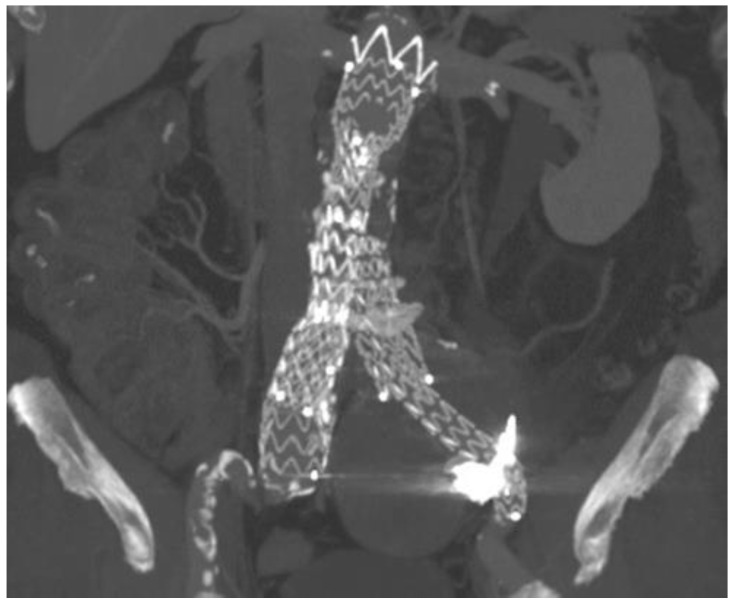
One-year enhanced CT scan showing left common iliac artery aneurysmatic sac stability and no endoleaks during the late contrast phase.
